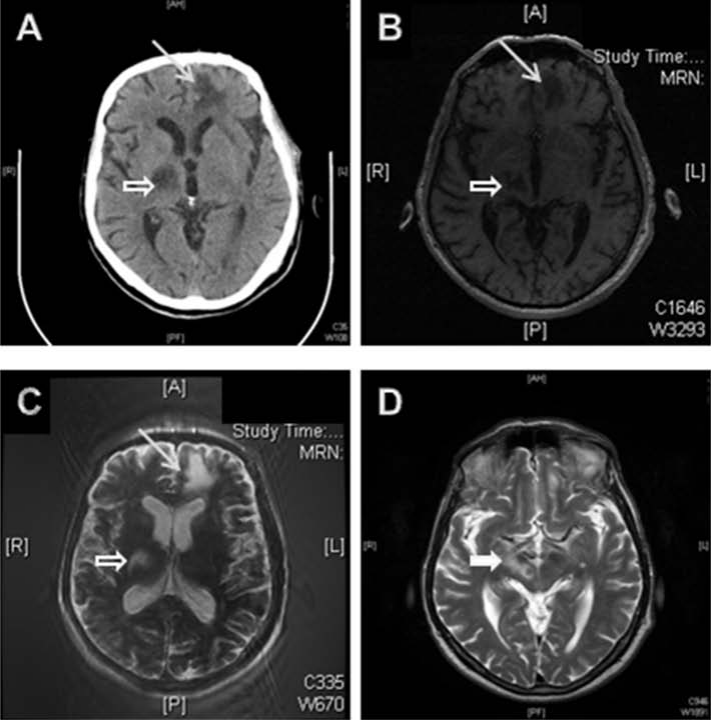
Imaging studies conducted in the final hospital. A, computed tomography of the head without contrast. B, T1-weighted magnetic resonance imaging of the head. C and D, T2-weighted magnetic resonance imaging of the head. The arrows indicate the subcortical lesion in the left frontal lobe; the block arrows indicate the lesion in the right posterior limb of the internal capsule and thalamus extending along the corticospinal tract (solid arrow, image D)
